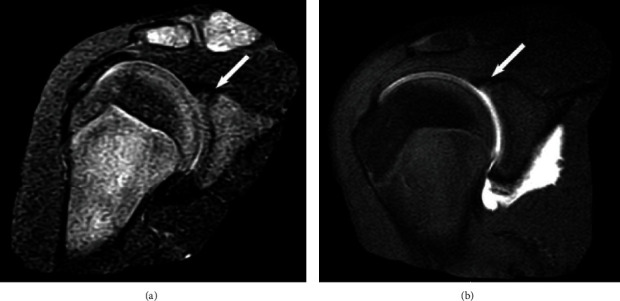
MR images of the patient's injured shoulder. Arrows point to the suspected SLAP tear in both images. (a) This image was taken approximately 11 weeks after the index injury. (b) This image is from the second MRI, which was approximately 27 weeks after the index injury. Because T2-weighted imaging was not performed during the second MRI scan, a direct comparison cannot be made to part (a). Also, the left (noninjured) shoulder was not evaluated with MRI.
